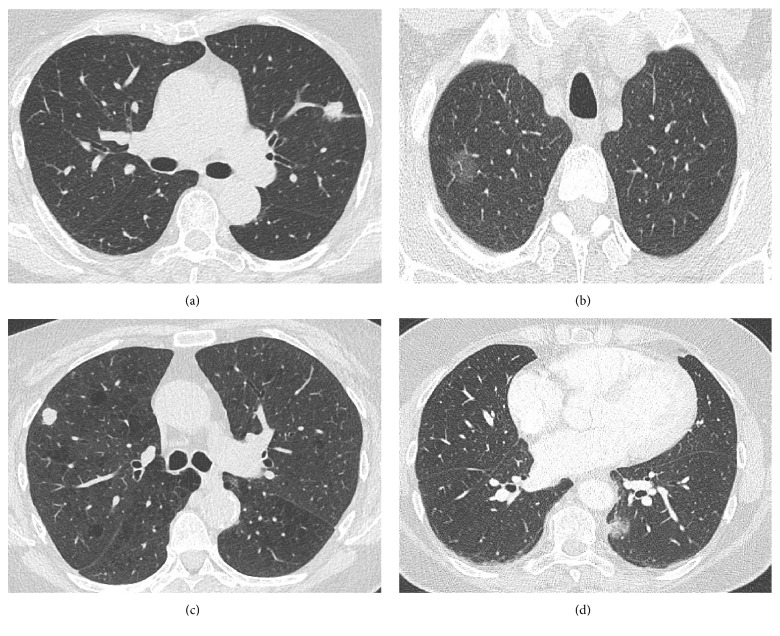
CT scans in four representative patients. Panel (a) presents a solid nodule of 16 mm in diameter in the left upper lobe in a 75-year-old woman. The final diagnosis was tuberculosis. Panel (b) presents a nonsolid nodule of 20 mm in diameter in the right upper lobe in a 57-year-old man. The final diagnosis was lung adenocarcinoma. Panel (c) presents a solid nodule of 11 mm in diameter in the right upper lobe in a 72-year-old woman. The final diagnosis was small cell lung carcinoma. Panel (d) presents a nonsolid nodule of 14 mm in diameter in the left lower lobe in a 67-year-old woman. The final diagnosis was lung adenocarcinoma.
